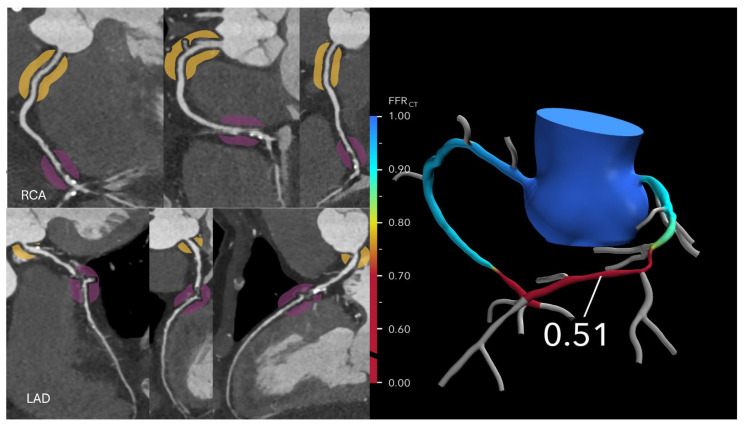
Optimizing treatment decisions with FFR-CT: (Left): A curved multiplanar reformatted coronary CTA image in a 64-year-old man with stable chest pain reveals severe stenoses in the mid left anterior descending (LAD) artery and distal right coronary artery. (Right): The corresponding FFR-CT image shows a value of 0.51 measured 2 cm distal to the lesion, indicating severely reduced flow. Based on these findings, the patient proceeded to invasive coronary angiography, followed by intervention. Please refer to Figure 4’s caption for the degree of stenosis in relation to different colors. Reprinted with permission under open access from Kasaeian et al. [6].
